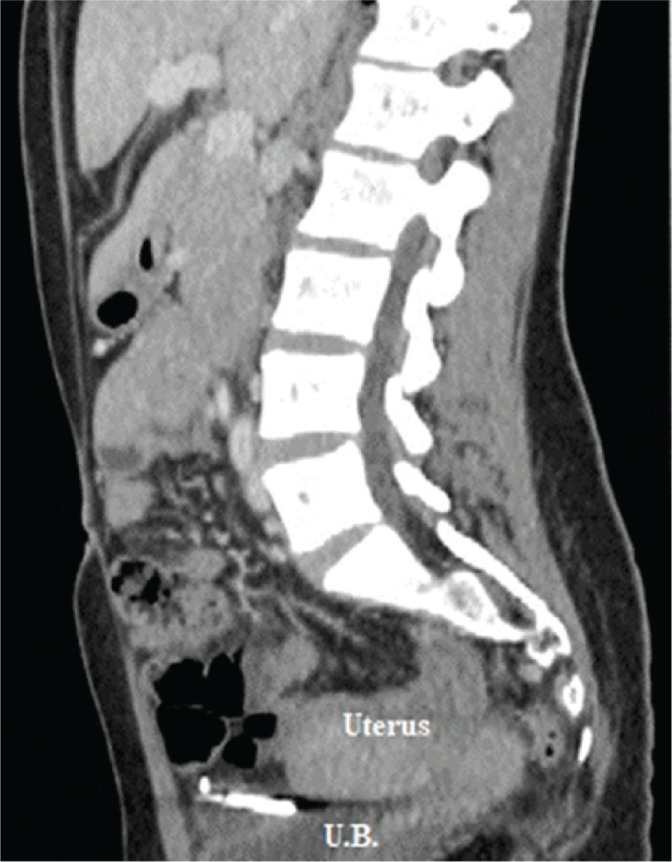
Computed tomography confirms an IUD to be extrauterine in location, between the urinary bladder and uterus.
